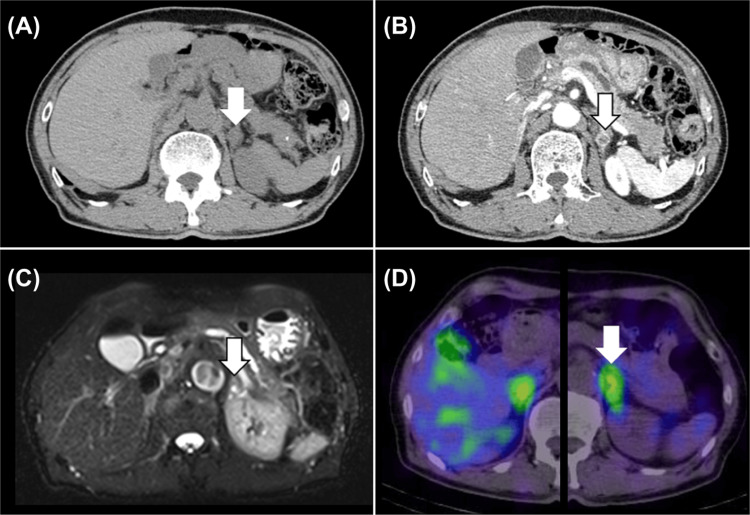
Imaging examination of the right adrenal tumor in case 47. (A) Plain CT. (B) Contrast-enhanced CT. (C) T2-weighted MRI. (D) 123I-MIBG scintigraphy. (A) Unenhanced CT image showing a small left adrenal lesion. (B) Contrast-enhanced CT demonstrating faint enhancement. (C) T2-weighted MRI exhibiting heterogeneous high signal intensity. (D) ¹²³I-MIBG scintigraphy demonstrated no laterality of uptake between the adrenal tumor and the contralateral adrenal gland, suggesting only physiological adrenal uptake. Therefore, the scan was considered negative for pheochromocytoma. ^123^I-MIBG : ^123^I-metaiodobenzylguanidine.
